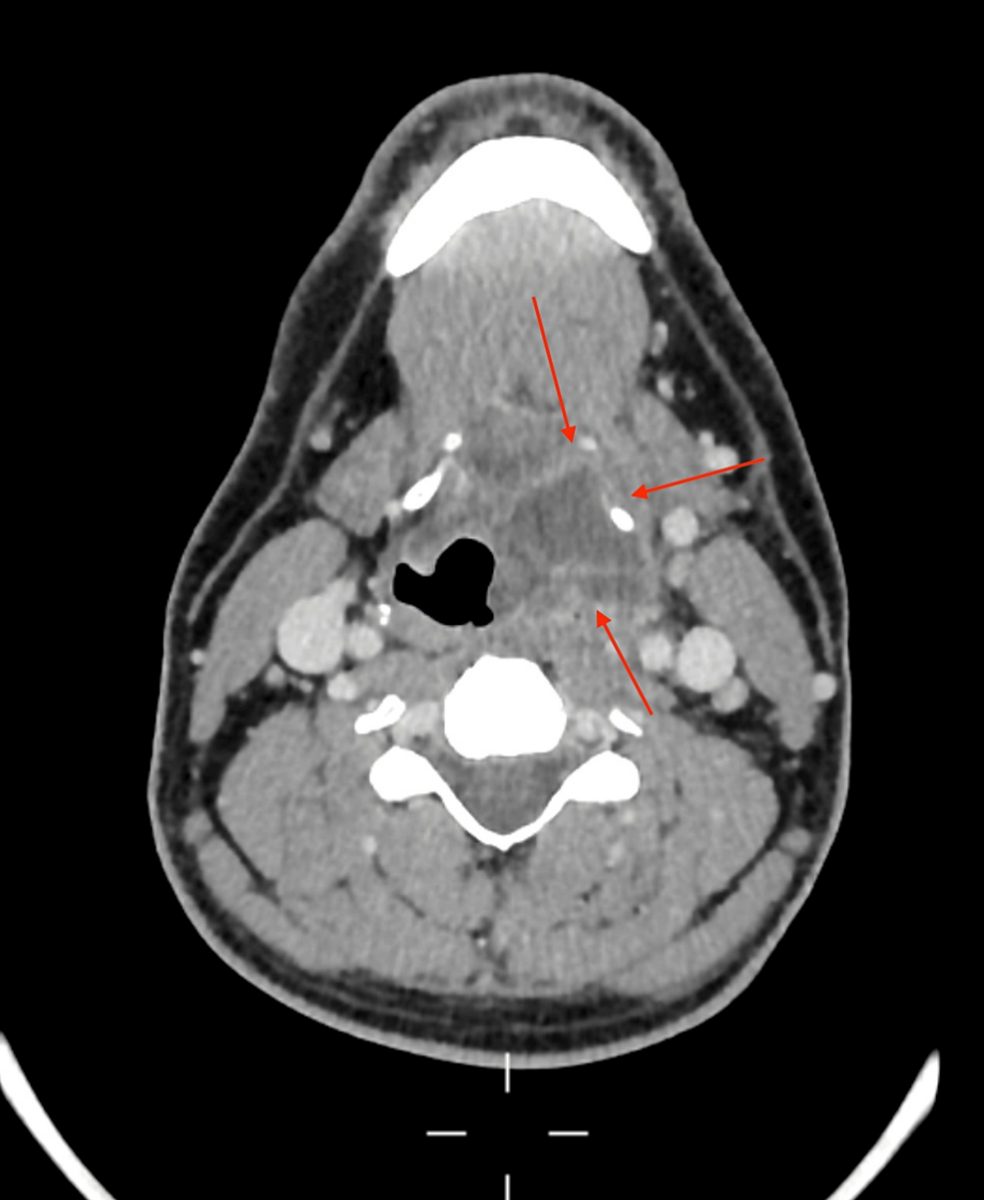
Axial view of a CT scan of the neck at the level of C1/C2 demonstrating a collection behind the left tonsillar fossa (red arrows)
